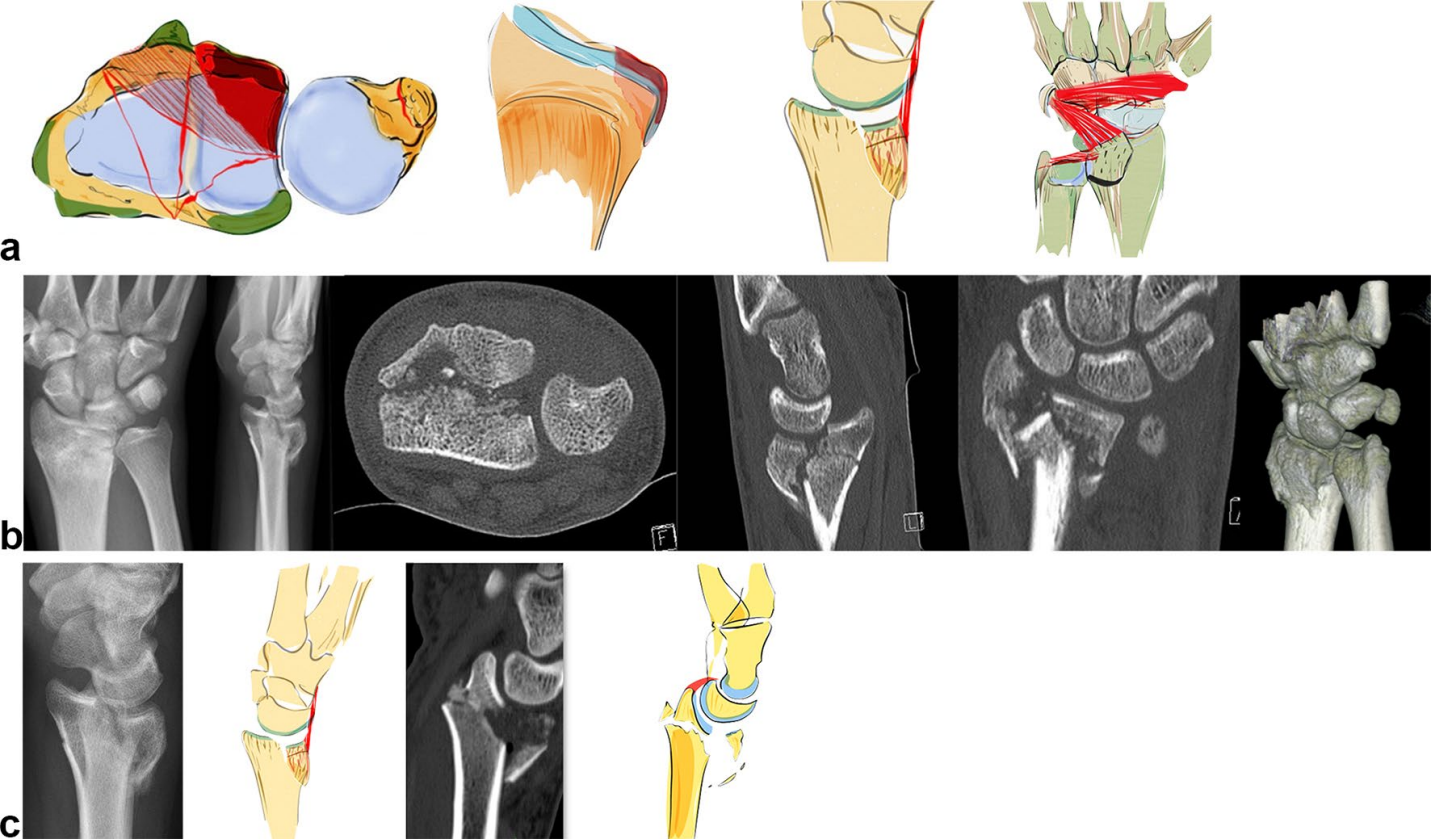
Dorsal key fragment: carpus dislocates with the key fragment to the dorsal side with dorsal extrinsic insertions. The palmar ligaments are ruptured (a). The plain X-rays show the grade of dislocation in a dorsal direction. On the CT scans, however, an additional depression of the articular surface, a step-off of the sigmoid notch and the dorsal key fragment is visible (b). A dorsal fragment does not necessarily have to be a key fragment. If the palmar ligaments are ruptured and the carpus and the dorsal fragment dislocate as a unit, the dorsal fragment has to be fixed first, as seen on the left two images. If, despite dorsal dislocation, the volar fragment is still attached to the palmar ligamentous apparatus (parallel articular lines between lunate and palmar articular surface), this palmar key fragment, comprising the osteoligamentous unit, requires special attention and has to be fixed first as seen on the right two images (c)
